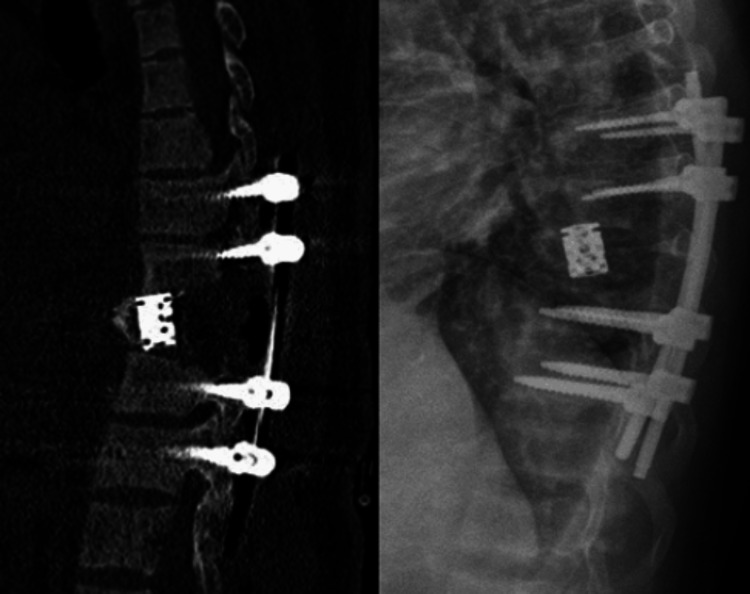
Postoperative CT and X-ray of the spine depicted adequate kyphosis correction and appropriate screw-rod construct positioning
